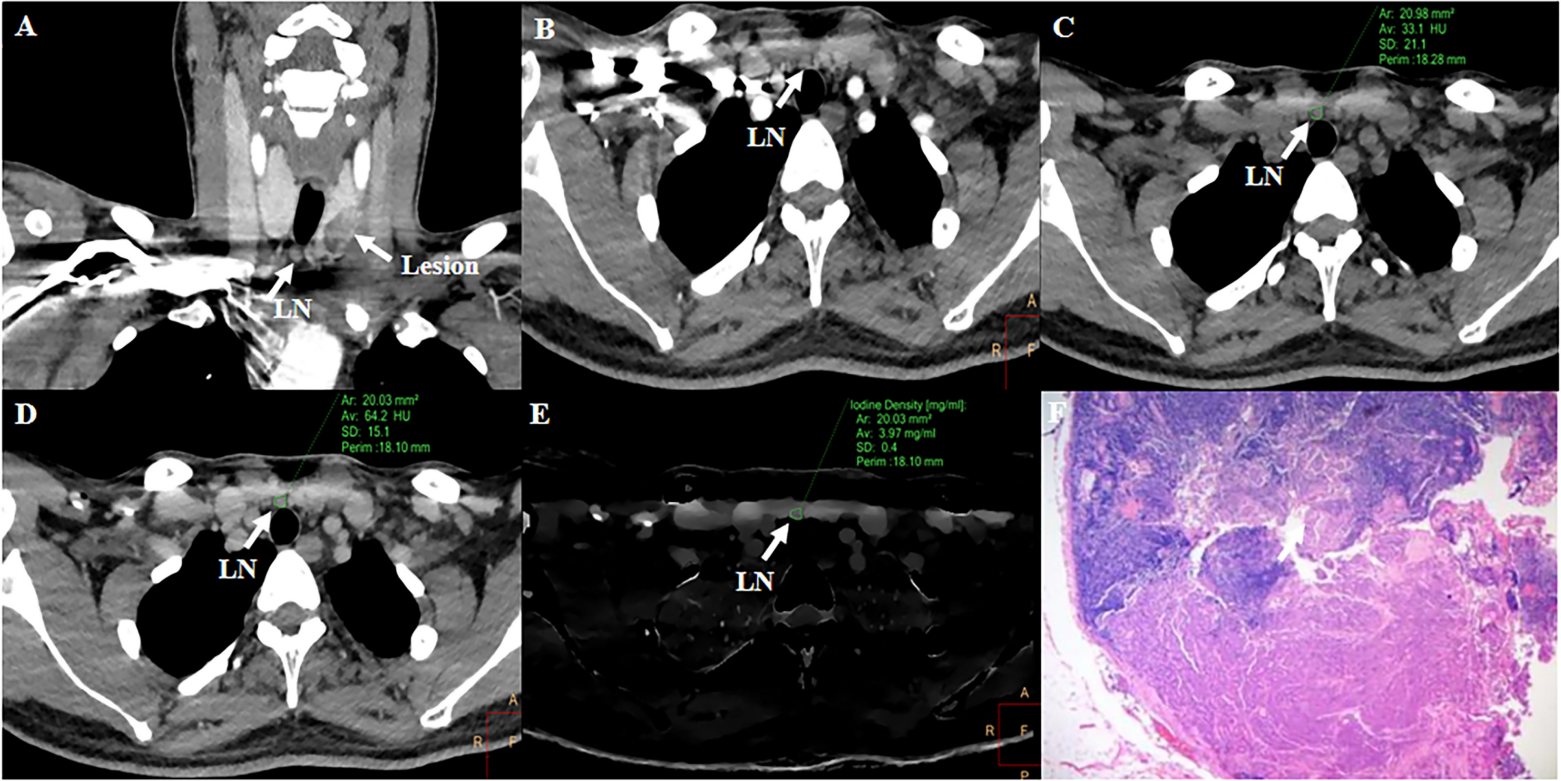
Representative case of using ECV_S_ and ECV_D_ to diagnose LN metastasis affected by cervical root artifact on arterial phase CT. A 36-year-old PTC patient with a LN in the right VI level. Right cervical root artifact existed coronal (A) and axial (B) early arterial phase CT images. Right cervical root artifact was reduced in equilibrium phase images. Attenuation values and iodine density were measured in the unenhanced (C), equilibrium phase 120-kVp equivalent blended image (D), and iodine map (E). The ECV_S_ and ECV_D_ of the LN were 50.32 and 53.15%, respectively. Subsequent pathological examination confirmed this LN as metastatic (F).
